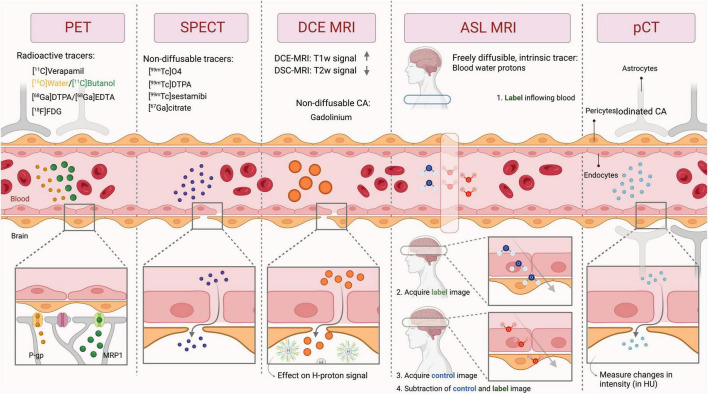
General principle of the imaging techniques used to measure BBB integrity. PET, Positron emission tomography; SPECT, Single-photon emission computerized tomography; DCE MRI, Dynamic contrast-enhanced magnetic resonance imaging; ASL MRI, arterial spin labeling MRI; pCT, perfusion computed tomography; CA, contrast agent; HU, hounsfield units; P-gp, P-glycoprotein; MRP1, multidrug resistance-associated protein 1; T1w, T1-weighted; T2w, T2-weighted.
